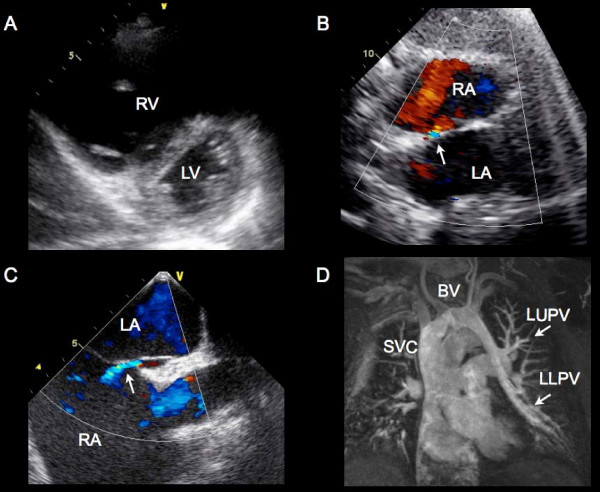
Multimodality imaging of anomalous pulmonary veins: A) A parasternal short axis view on TTE demonstrating moderate RV enlargement. RV, right ventricle; LV, left ventricle. B) A subxiphoid view on TTE demonstrating left to right shunting across the interatrial septum on color Doppler, consistent with a PFO. RA, right atrium; LA, left atrium. C) A midesophageal view on TEE demonstrating left to right shunting across the interatrial septum on color Doppler, consistent with a PFO. RA, right atrium; LA, left atrium. D) Cardiac magnetic resonance imaging (CMR) demonstrated a congenital partial anomalous connection of both left pulmonary veins to the innominate vein. SVC, superior vena cava; BV, brachiocephalic vein; LUPV, left upper pulmonary vein; LLPV, left lower pulmonary vein.
